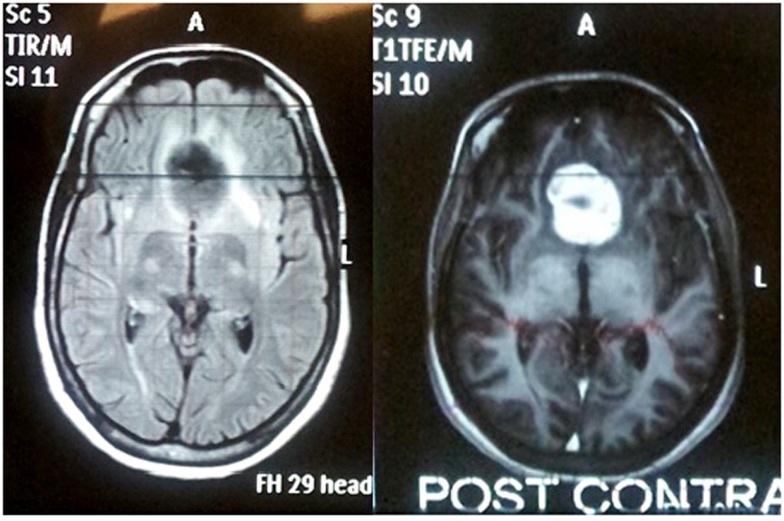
MRI of the brain with and without contrast done in Rwanda 1 year previous to admission to our institution showed 3 cm × 2.6 cm × 1.2 cm homogeneously enhancing suprasellar mass along the dural floor of the cranial fossa.
